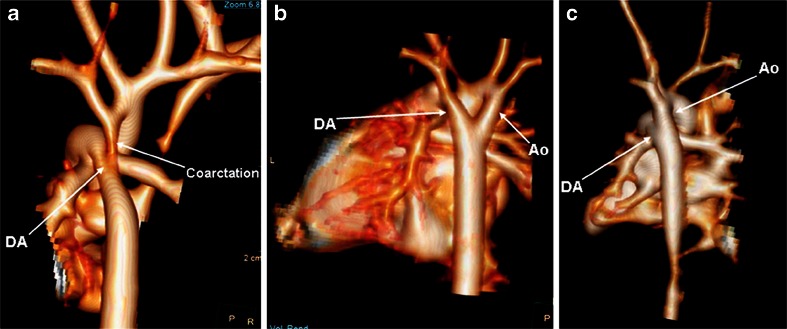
Fetal postmortem CT angiography of arch abnormalities. Postmortem CT angiography with 3-D visualization shows (a) coarctation of the aorta in a fetus at 24 weeks’ gestation, (b) right aortic arch in a fetus at 25 weeks’ gestation, and (c) normal great vessels in a fetus at 25 weeks’ gestation. *Ao* aorta, *DA* ductus arteriosus. Reproduced with permission [17]
